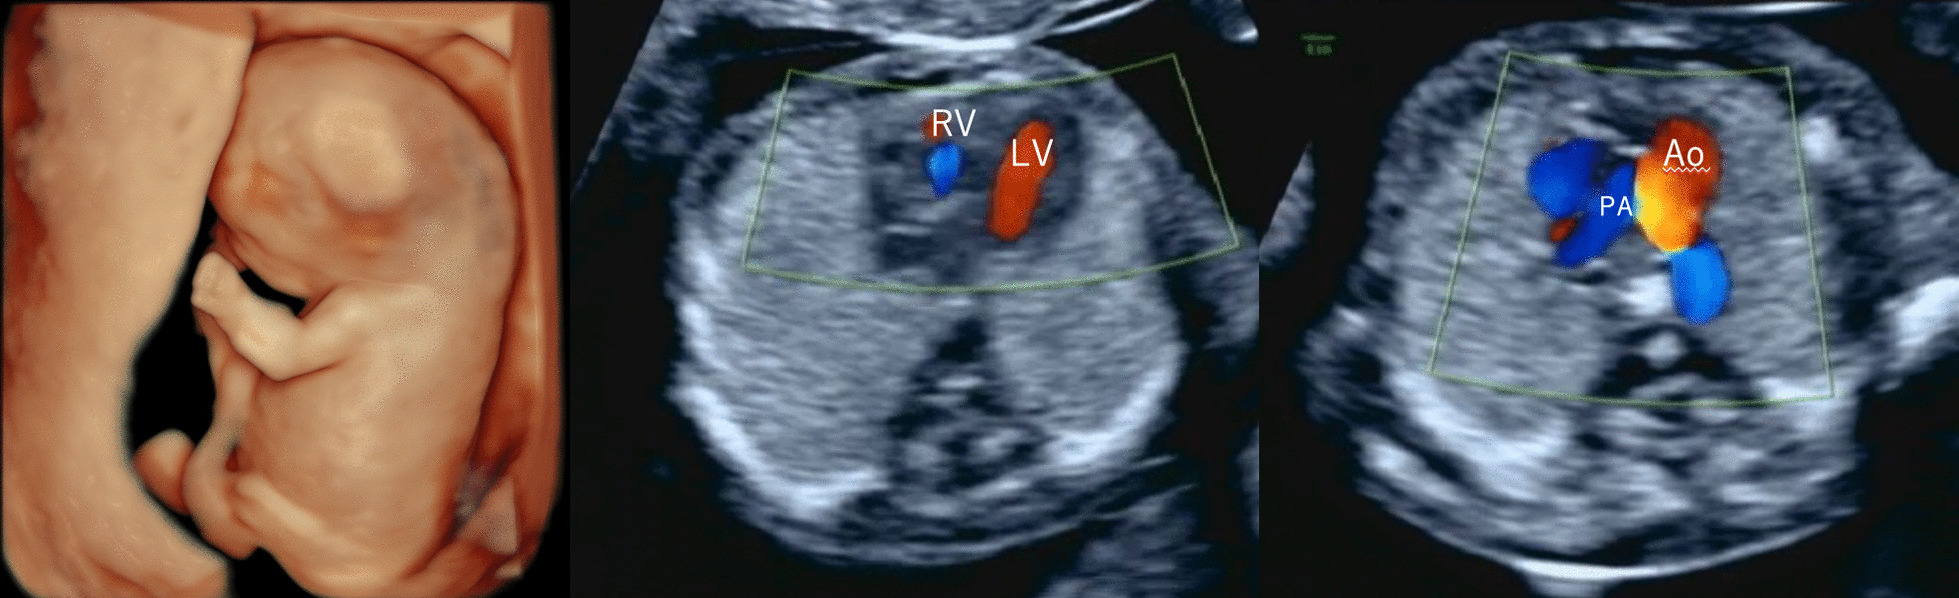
Fetal ultrasound images; 11 weeks and 5 days of gestation in the third pregnancy. Fetal ultrasonography showed mild fetal hydrops (the left image), moderate tricuspid valve regurgitation (the central image), and decreased right ventricular contractility (the right image)
